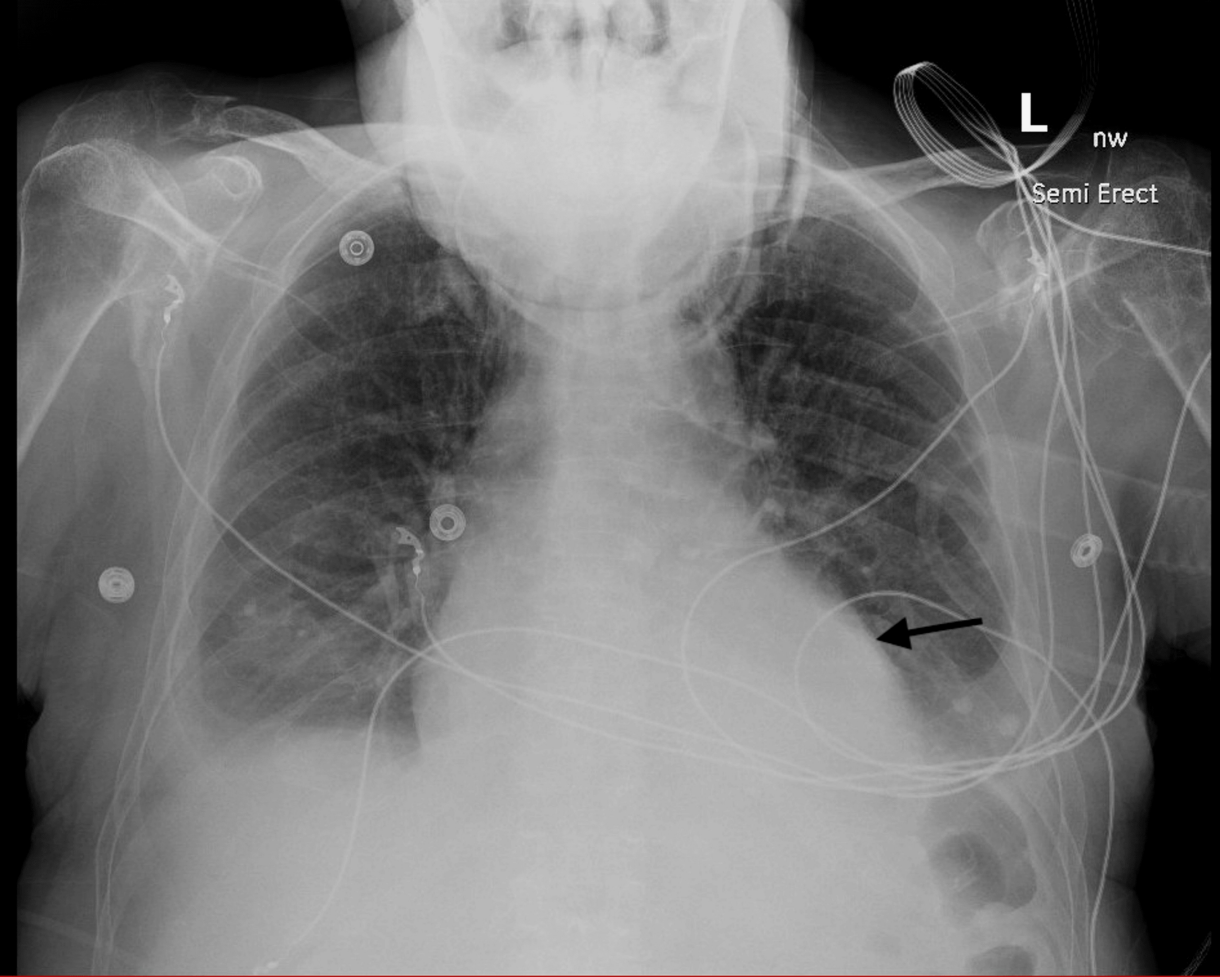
Semi-erect chest X-ray showed pericardial effusion.
